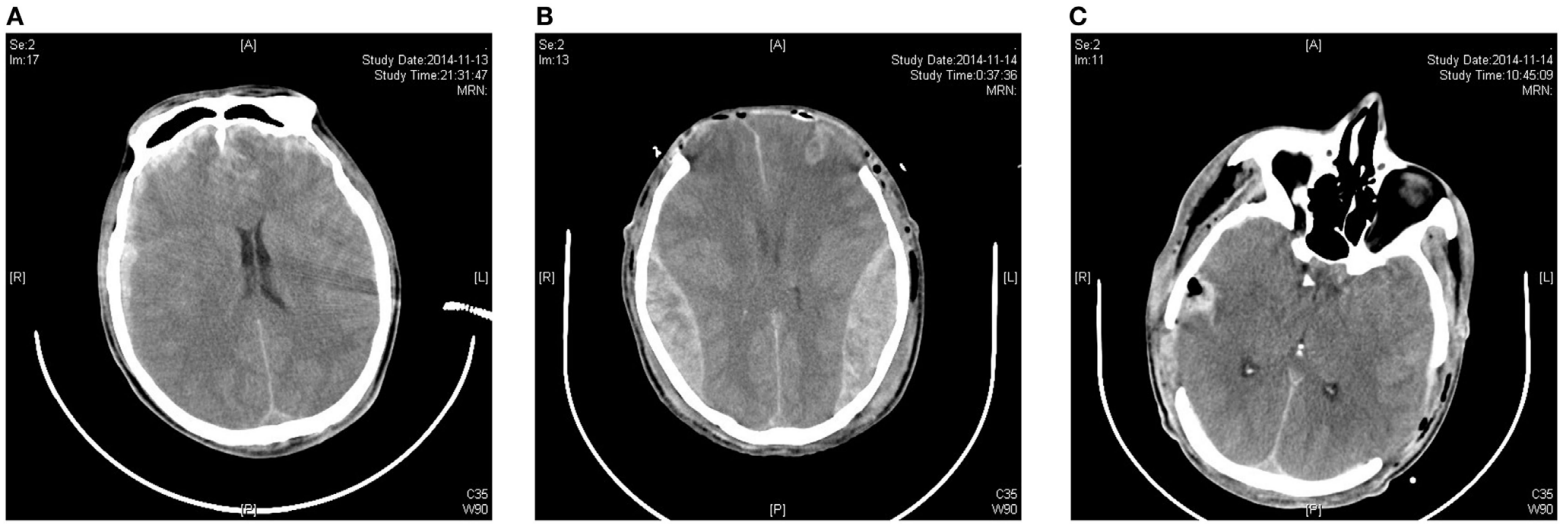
A rapid decompression-induced acute encephalocele further induces the occurrence of the contralateral epidural hematoma. (A) Preoperative CT skull hints double frontal cerebral contusion; (B) postoperative CT skull hints the bilateral temporal occipital epidural hematoma; (C) postoperative CT skull hints bilateral epidural hematomas completely cleared.
